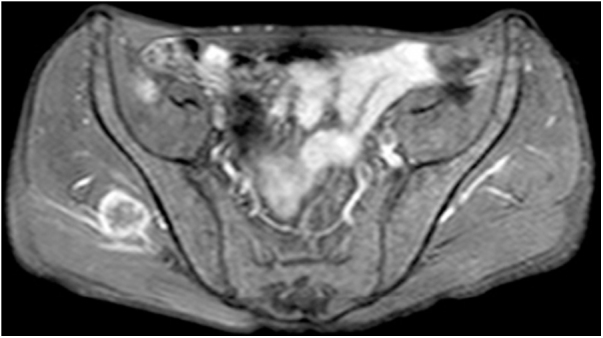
MRI evidence of right gluteus medius muscle metastasis.
